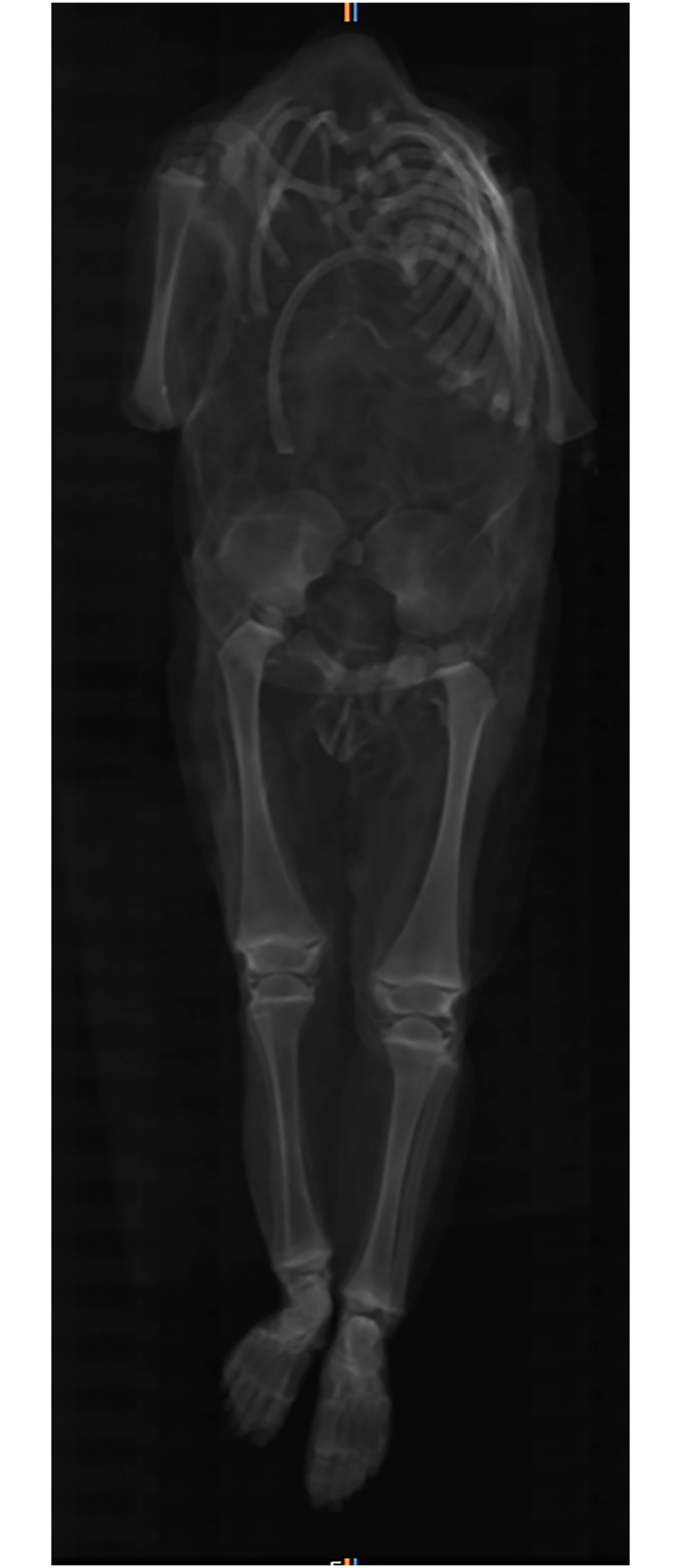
Coronal multi-planar reconstruction of a CT data set showing individual G. The trunk skeleton is largely disintegrated. Note the presence of the ossification centres of the proximal humeral head, proximal femoral head and distal femur, proximal and distal tibia, and distal fibula.
